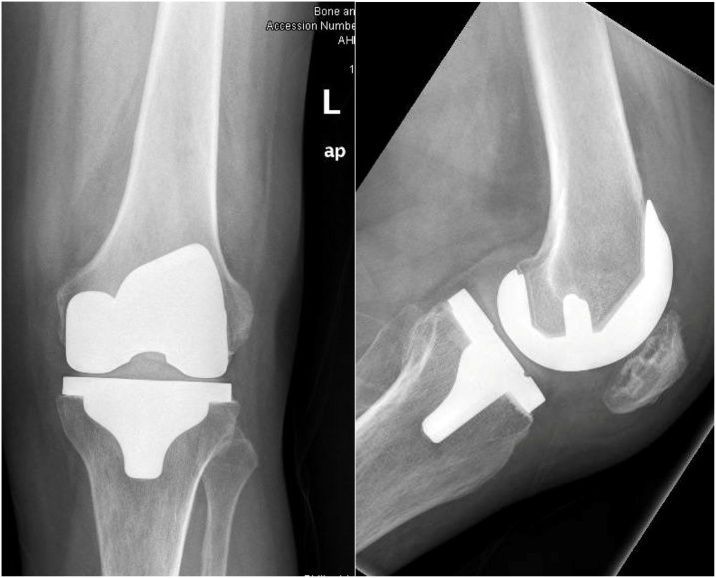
Postoperative X-ray showing cruciate retaining total knee arthroplasty.
